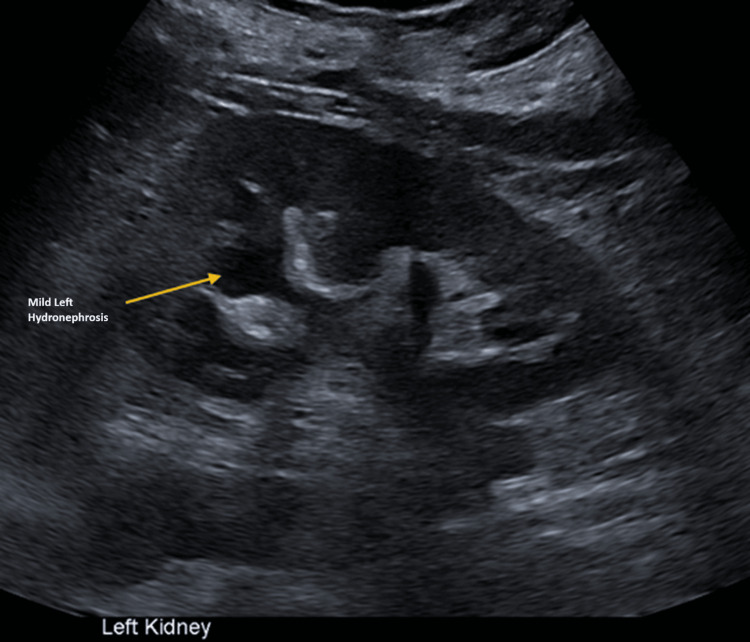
Ultrasound image of the left kidney demonstrating mild pelvicalyceal dilatation (hydronephrosis) (yellow arrow)
